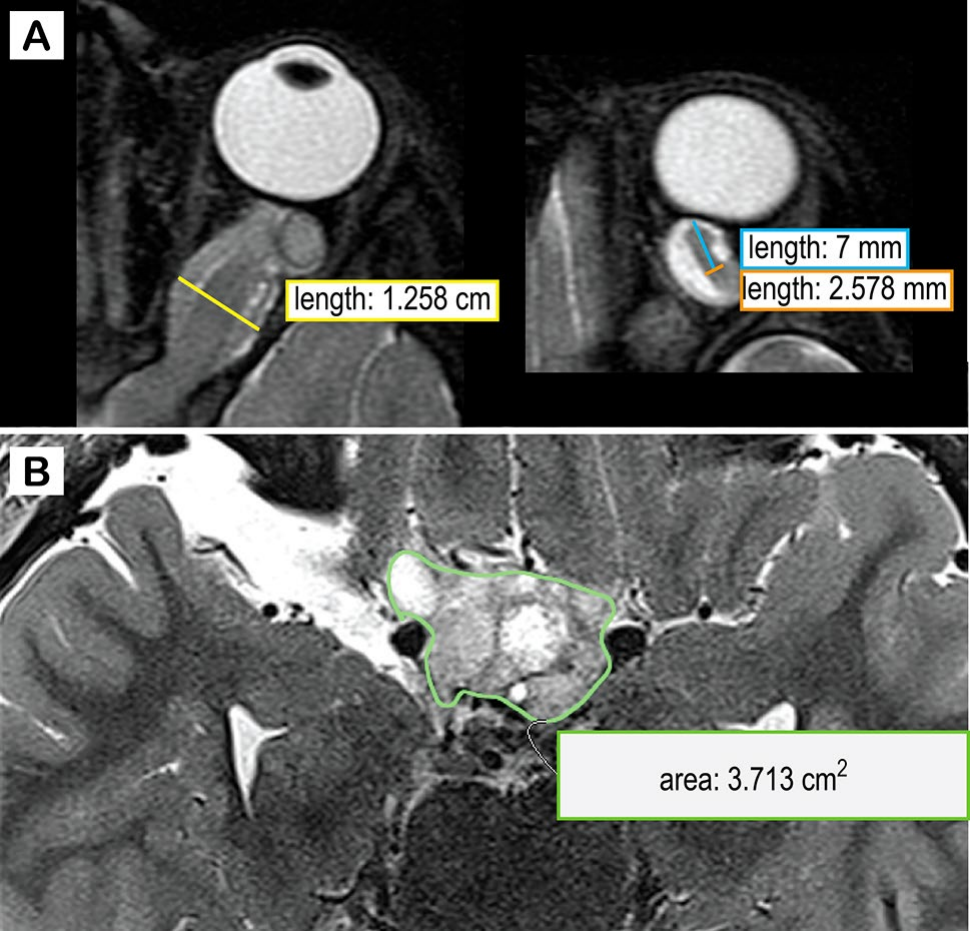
Assessment of tumor load. T2-weighted image of a 3 years old boy with neurofibromatosis type 1 and an optic pathway glioma. The optic nerve width was measured at its maximal width (yellow line in A) and 7 mm behind the globe (orange line in A). The intracranial tumor volume was determined by outlining the lesion on consecutive slice using the open source medical viewer Horos (Nimble Co LLC d/b/a Purview in Annapolis, MD USA). The inbuilt algorithm was used to calculate the overall tumor volume on the basis of the area and the slice thickness
